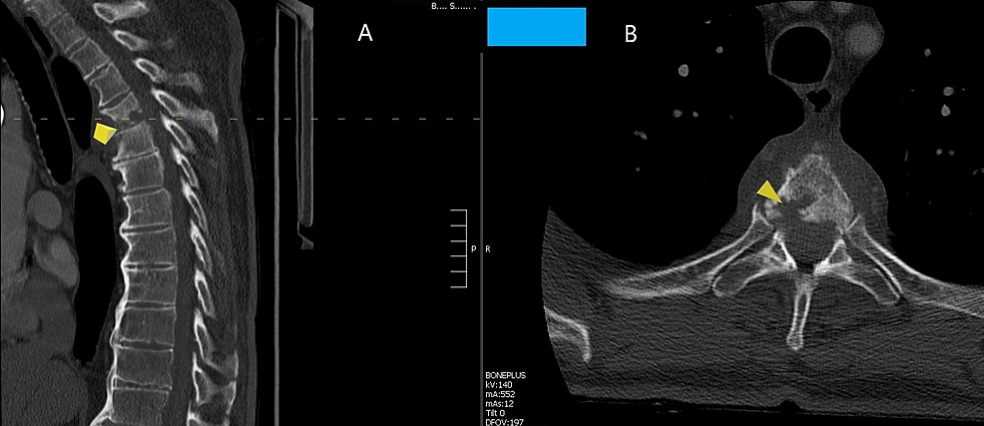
Preoperative CT image sagittal and axial of the thoracic spine A: Sagittal CT image revealing loss of disc height at T4-T5 with erosive changes of the end-plates and the vertebral bodies (marked by yellow arrow) B: axial image revealing erosion and loss of bone in the vertebral body at L4 more on the right side (marked by yellow arrow)
